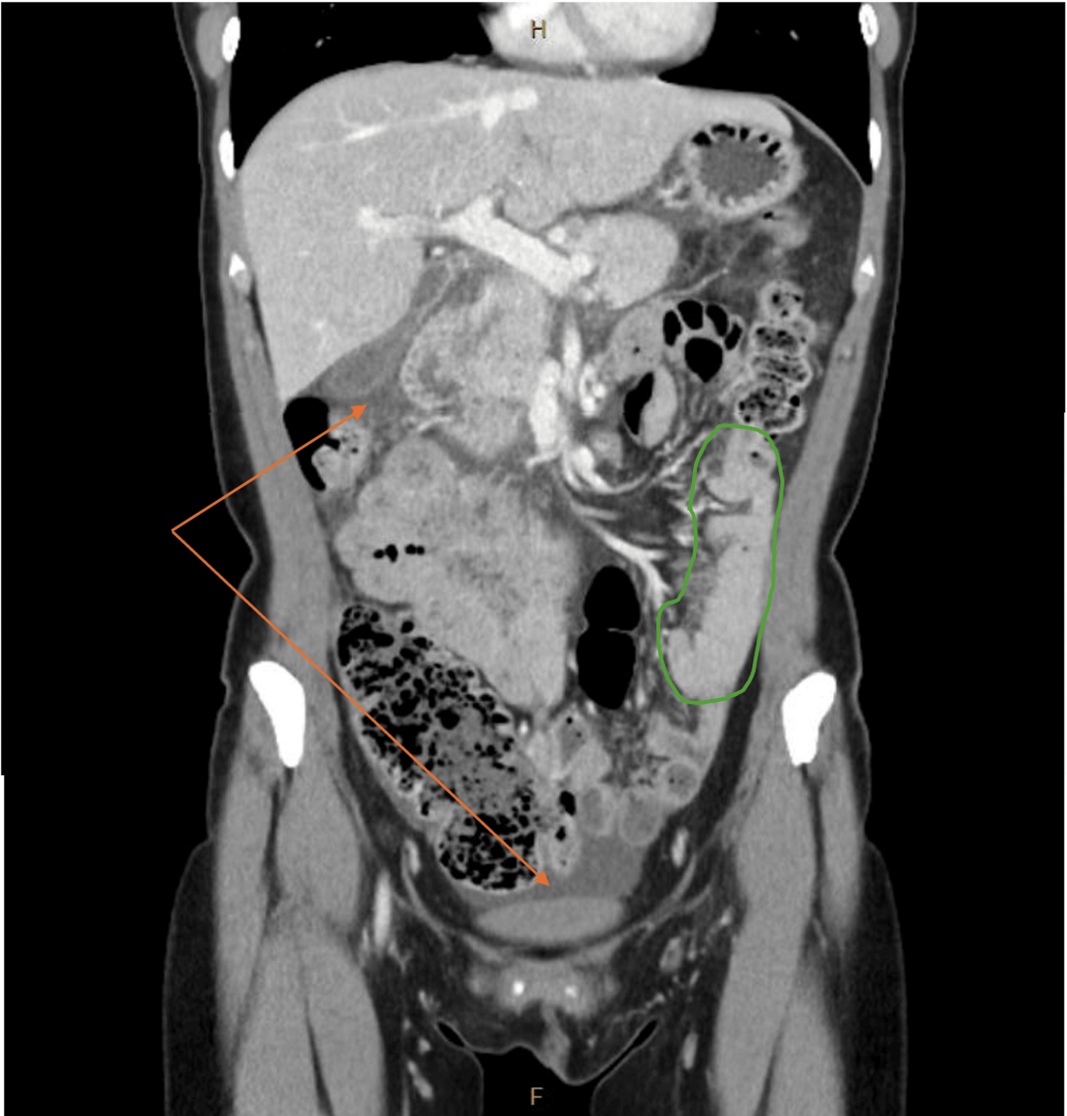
CT of the abdomen and pelvis (coronal view) in portal venous phase demonstrating cluster of displaced small bowel (outlined in green) and intraperitoneal free fluid (orange arrows)
